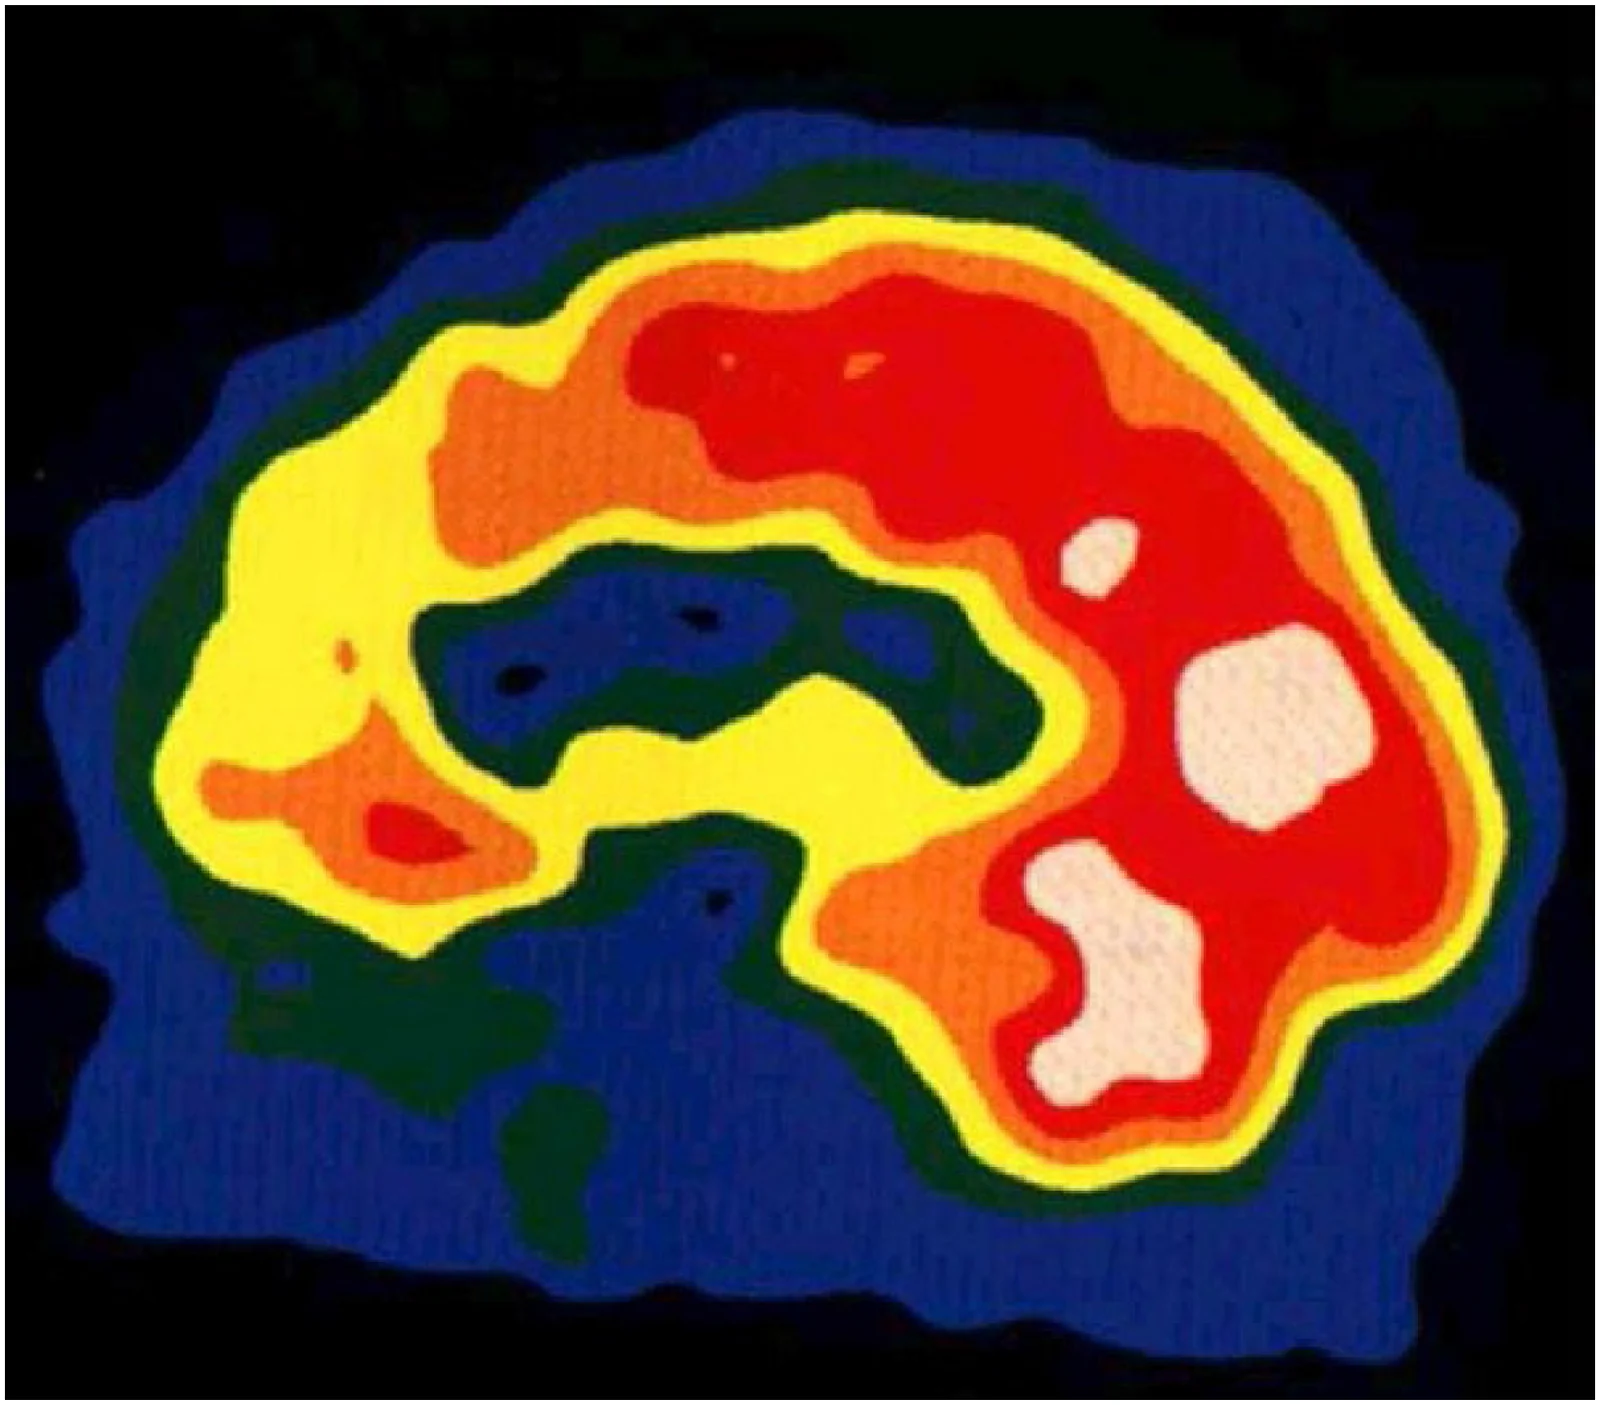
Cerebral SPECT reveals moderate to severe hypoperfusion in the frontal lobe.
